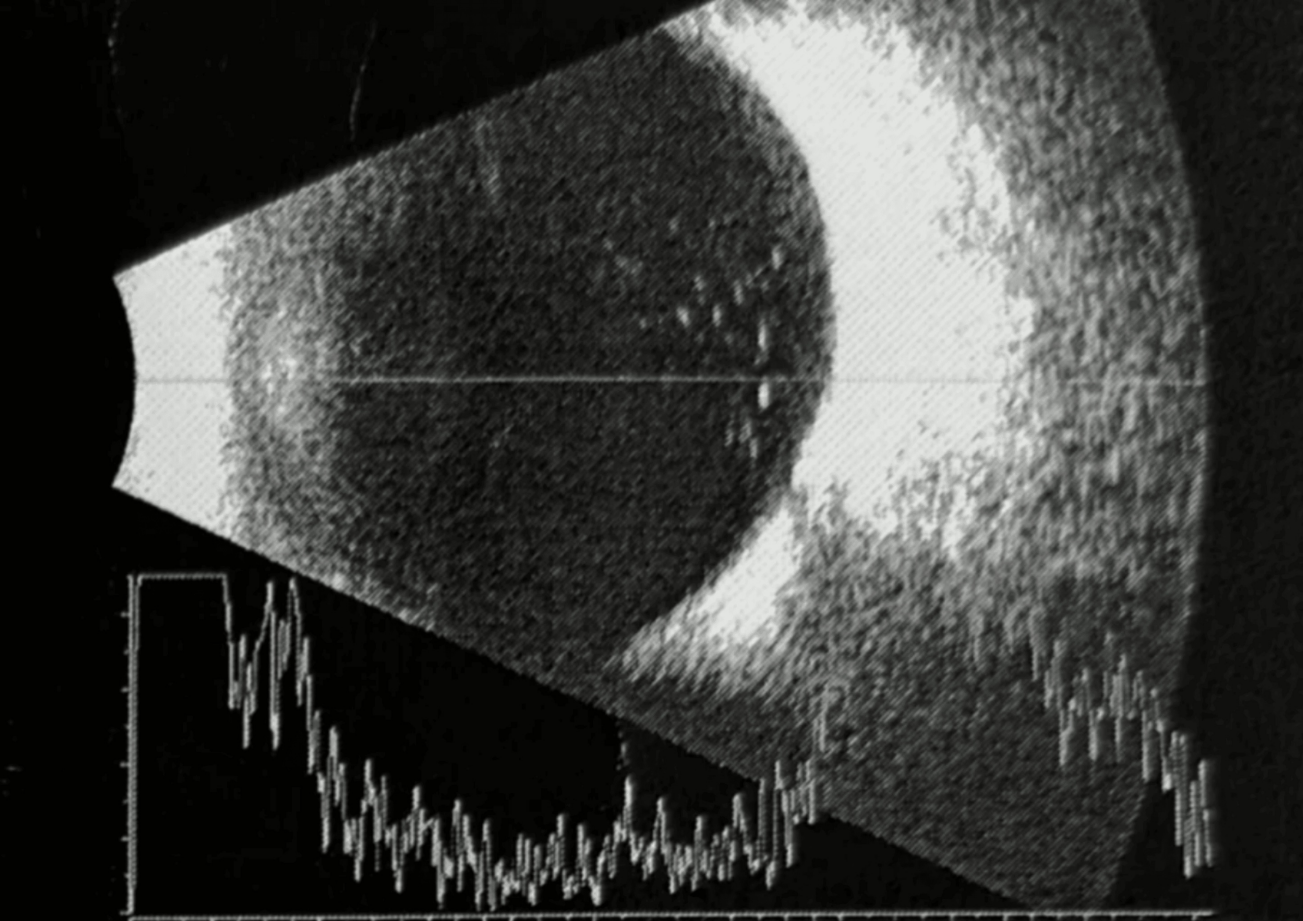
B-scan Ultrasonography of the Globe Demonstrating Preserved Posterior Segment Anatomy
